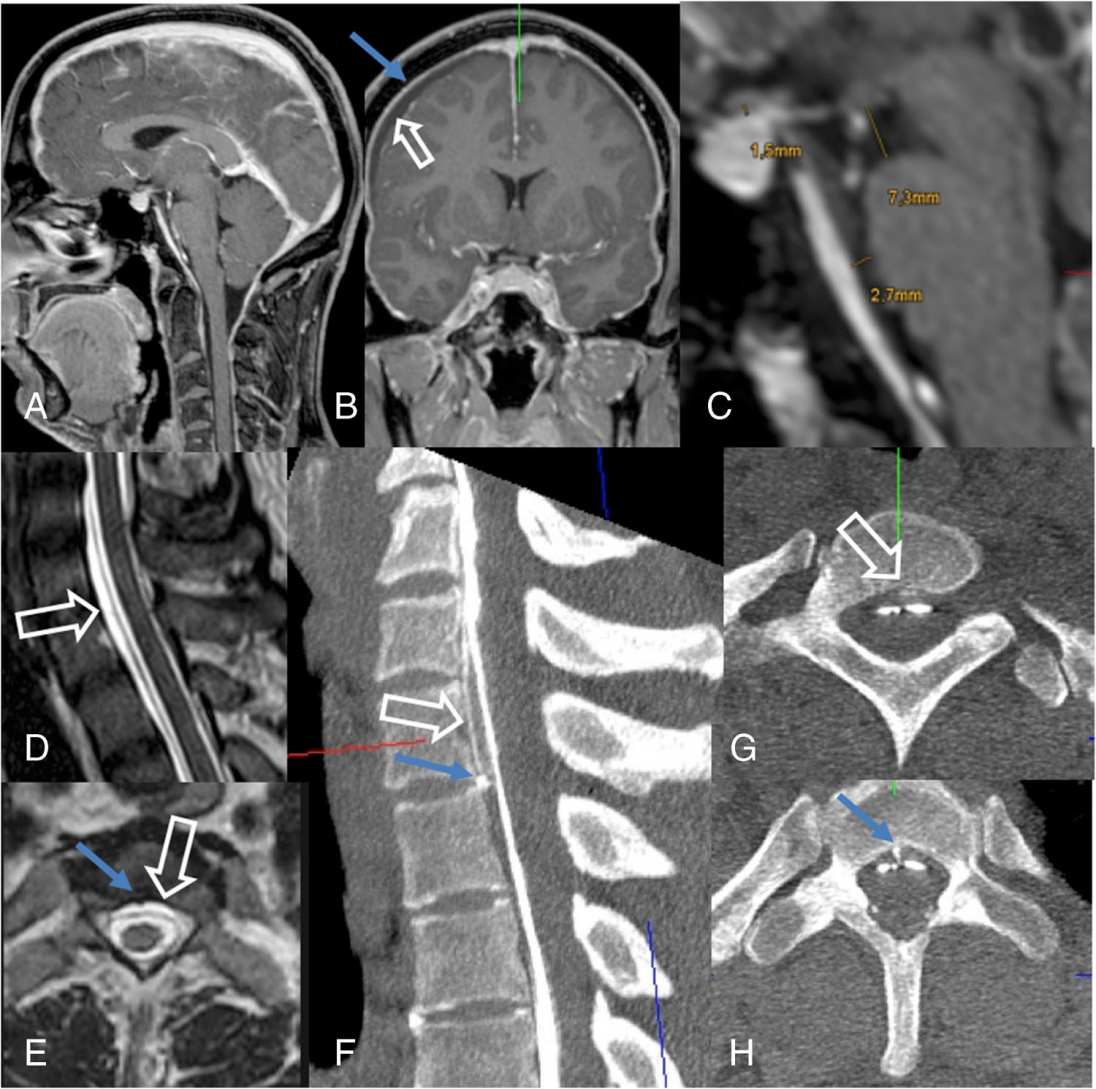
Type 1 leak: SIH in a 36-year-old woman with engorgement of the venous sinuses, pachymeningeal enhancement (B: arrow), subdural fluid collections (B: hollow arrow), effaced suprasellar (C: 1.5 mm), and prepontine cisterns (C: 2.7 mm). The mamillopontine distance is normal (C: 7.3 mm). Spinal MRI shows spinal longitudinal extradural fluid (SLEC) (D, E: hollow arrow). Prone dynamic CT myelography shows the ventral leak due to a bony spur (H: arrow) and ventral epidural contrast flowing upwards (F, G: hollow arrow)
